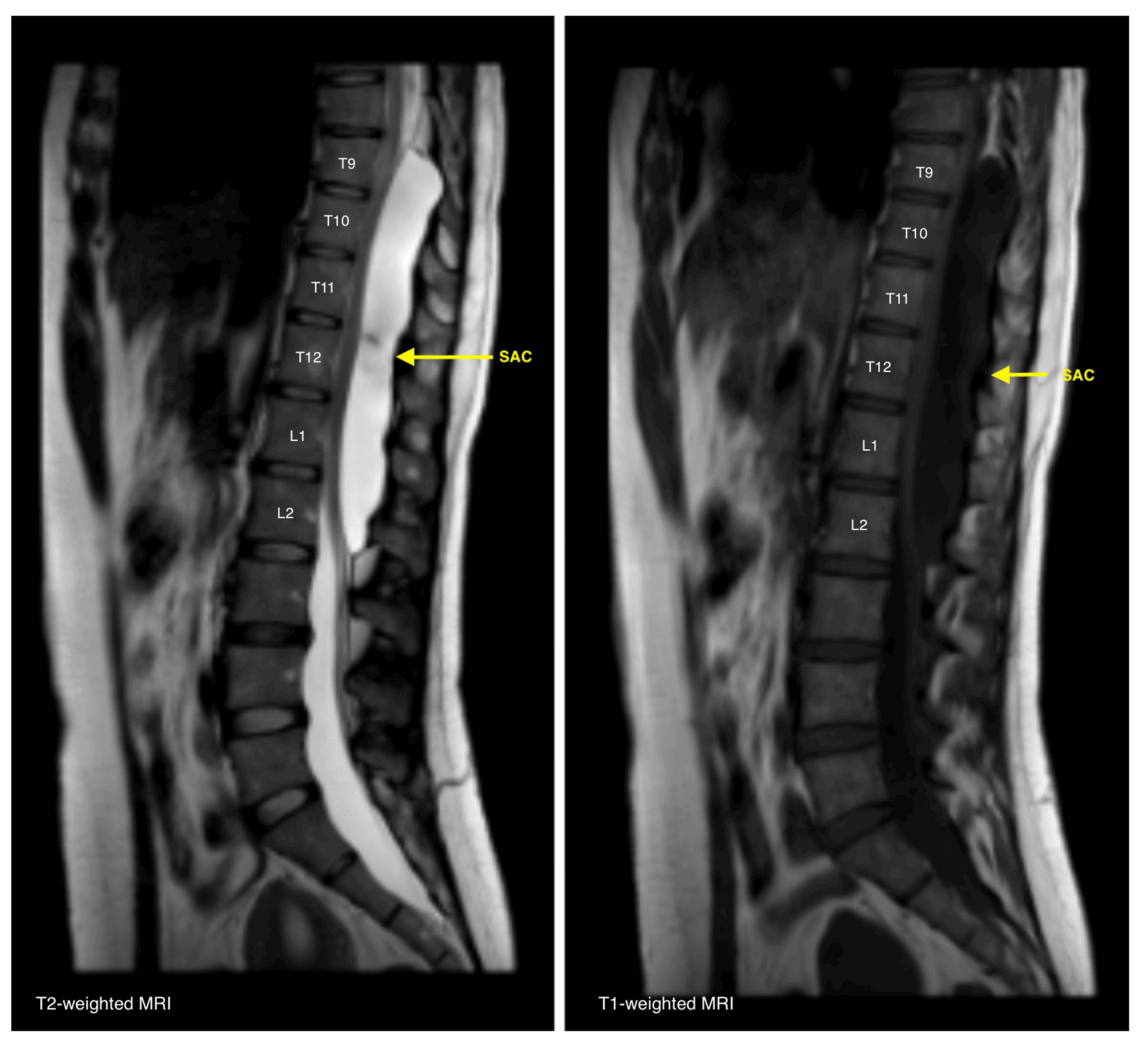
Preoperative sagittal T2-weighted magnetic resonance imaging (MRI) of the thoracolumbar spine demonstrating an extramedullary, extradural cystic lesion extending from T9 to L2 The lesion appears isointense to cerebrospinal fluid and causes anterior displacement of the spinal cord.
